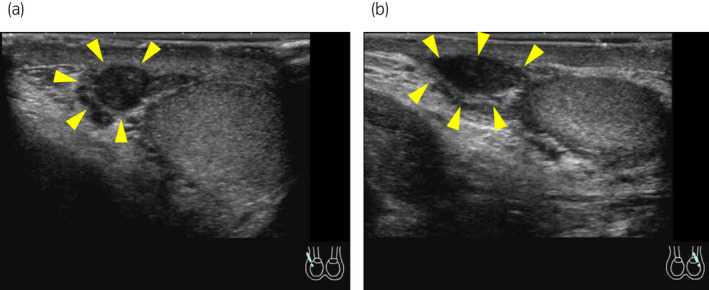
Ultrasonography of the scrotum (a, right; b, left) showed bilateral spindle‐shaped low echoic lesions located above the respective bilateral testes and epididymides (arrowheads), which had blood‐flow signals inside. It also detected normal but slightly small bilateral testes (a, right, 3.3 × 1.5 cm; b, left, 3.7 × 1.4 cm).
